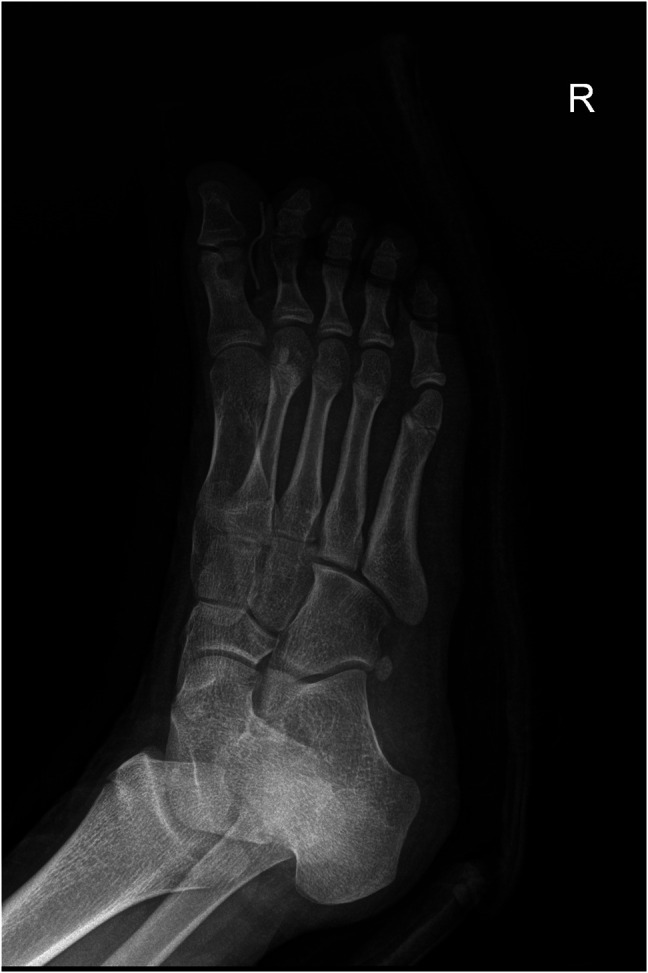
Immediate postoperative radiograph indicating complete resection of the bone tumor in the proximal phalanx of the great toe.
